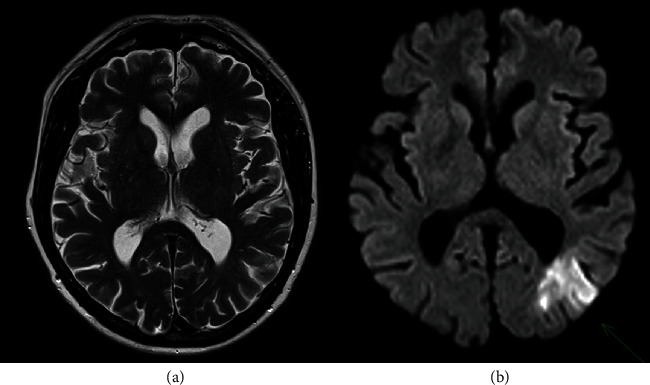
Axial diffusion-weighted brain MRI (b) demonstrating diffusion restriction involving the left occipital lobe at the junction of the left posterior cerebral artery and left middle cerebral artery territories (indicated by green arrow). Axial T2-weighted brain MRI (a) was unremarkable and did not demonstrate evidence of haemorrhagic transformation or previous stroke.
